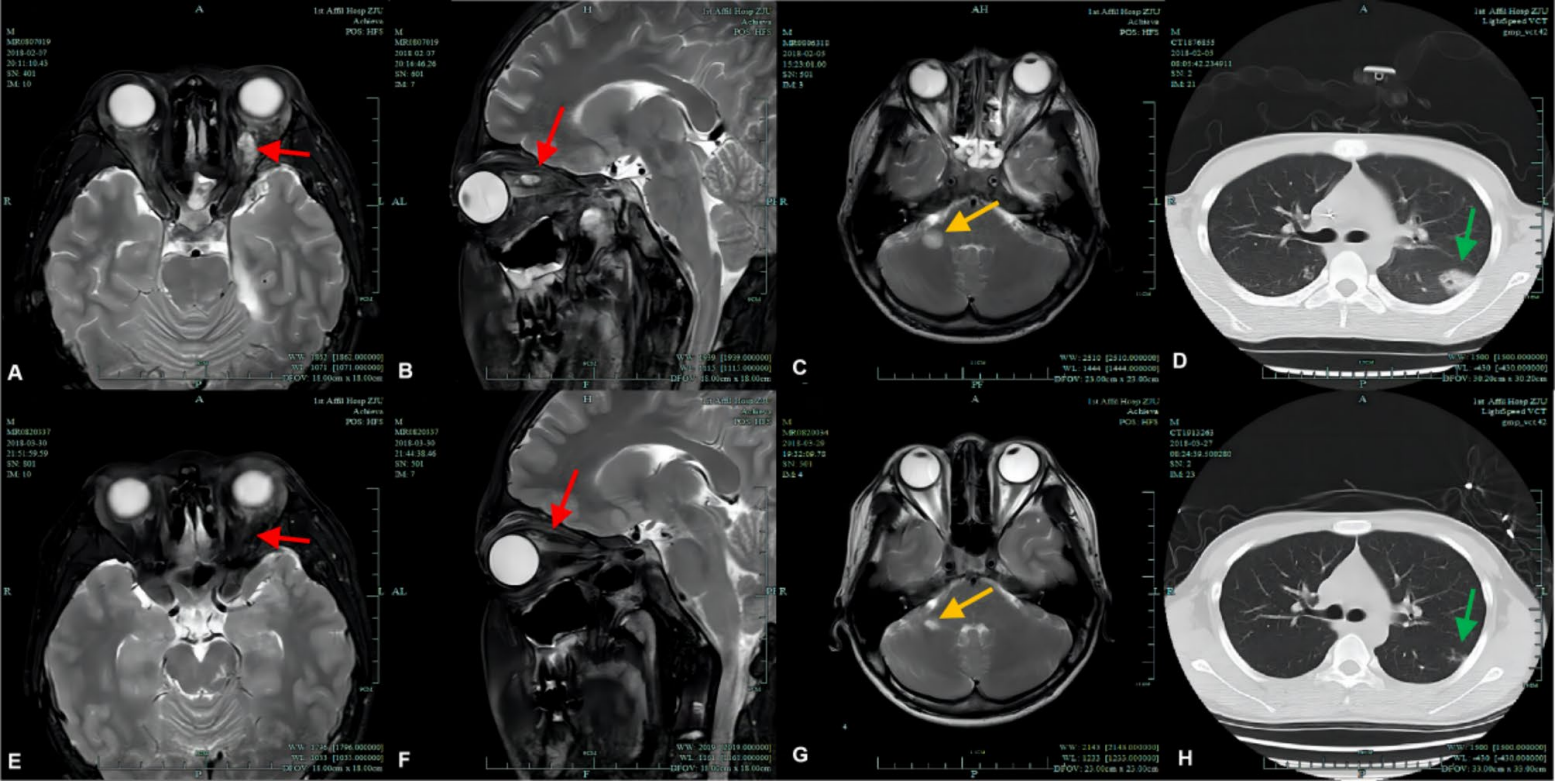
Orbital MRI, head MRI, and lung CT of case 2 (A, B, C, and D) were examined on early admission and (E, F, G, and H) were re-examined 50 days later before discharge. (D) Lung CT showing bilateral lung inflammation, which was more severe on the left side (green arrows). (C) Head MRI (T2, coronal) showing brain abscess formation (yellow arrows). (A,B) Orbit MRI (T2, coronal; T2, sagittal) showing bilateral orbital inflammation and abscess formation in the upper left orbit (red arrows). (E, F, G, and H) Follow-up MRIs and CTs showing that the inflammation and abscesses in the lungs, cerebrum, and orbits had mostly resolved before discharge
